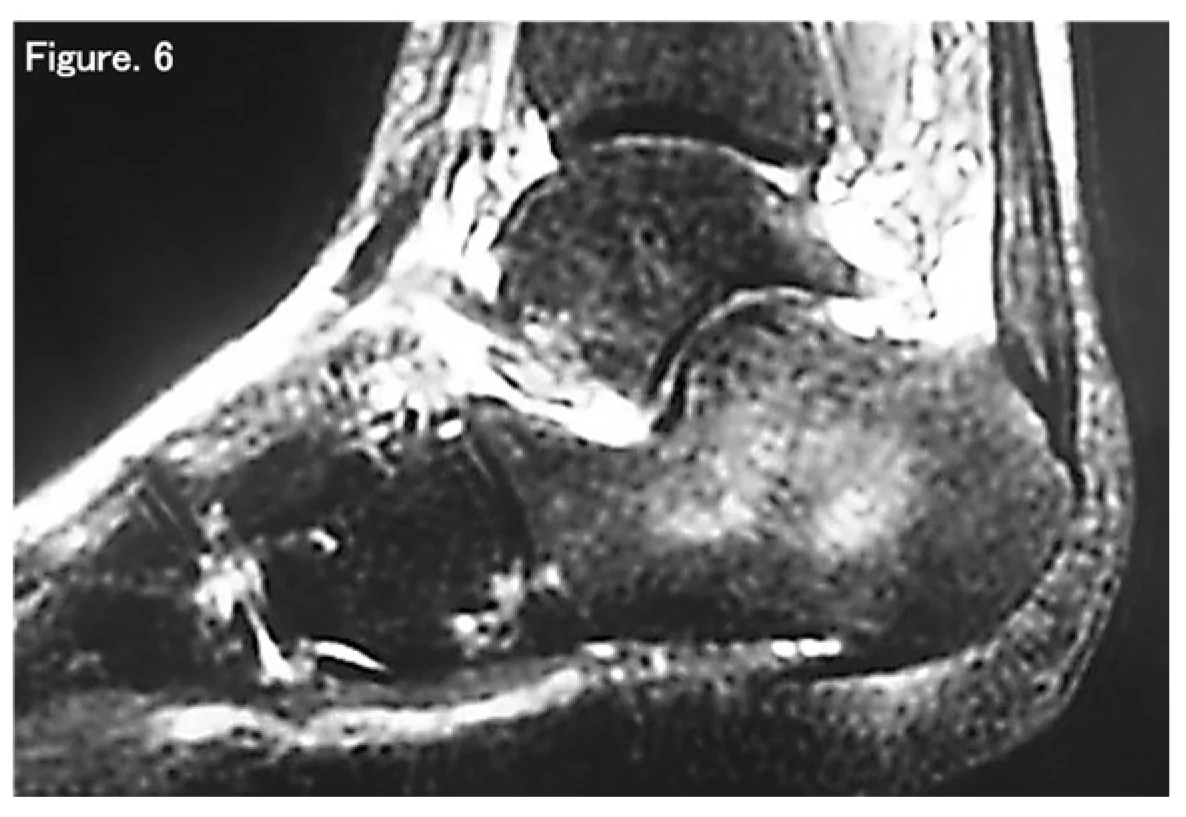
Sagittal fat-suppressed T2-weighted MRI of the same patient showing corresponding high-signal marrow oedema consistent with calcaneal insufficiency fracture.
